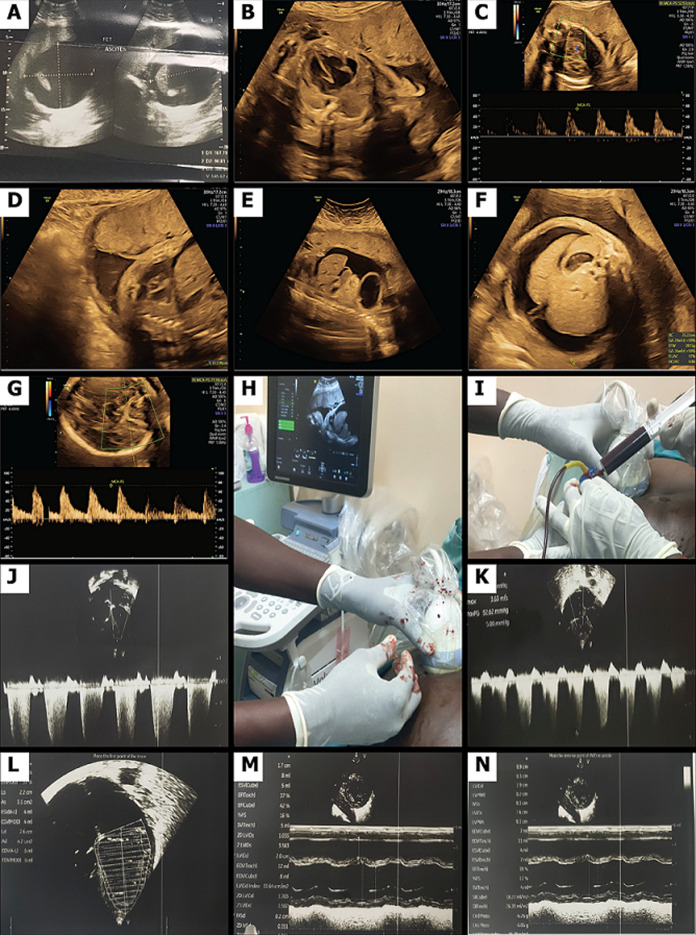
ultrasonographic findings and interventions across gestations, and postnatal echocardiographic findings (case one); A) massive fetal ascites at 27+5 weeks gestation; B-E) concurrent ultrasonography and fetal echocardiography at 30+0 weeks, showing cardiomegaly, pericardial effusion, thinning of the ventricular walls, and an MCA-PSV of 52.93 cm/s (1.39 MoM); F, G) progressive abdominal distension and skin edema consistent with worsening hydrops fetalis at 32+4 weeks; H, I) third ultrasound-guided intrauterine transfusion performed at 33+1 weeks; J, N) postnatal echocardiographic findings in the neonate
